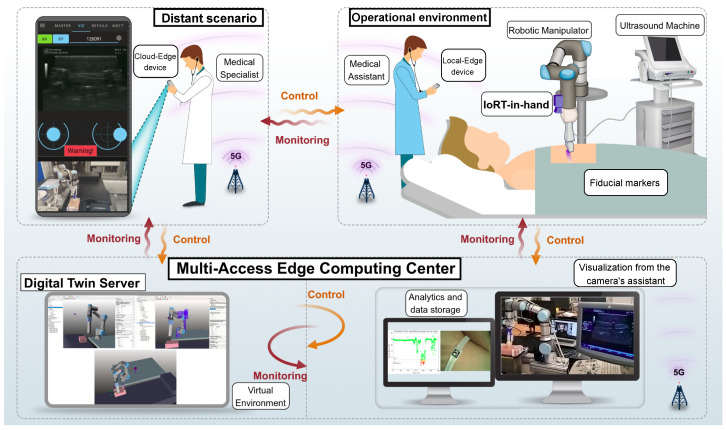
IoT application for ultrasound scanning in a geographically isolated health center. The patient needs to be diagnosed by a medical specialist who is not available in the area. A tele-robotic echography is performed by the specialist using the proposed open-source app to control the robot while performing the remote diagnosis. A view of both the virtual and real environments is available on the smartphone screen since the smartphone can access to the DT hosted on an MEC center and to the images provided by the RGB camera on the IoRT-in-hand. Analytics and data storage are implemented in the Cloud. A high-bandwidth and low-latency network is chosen and setup for the architecture.
